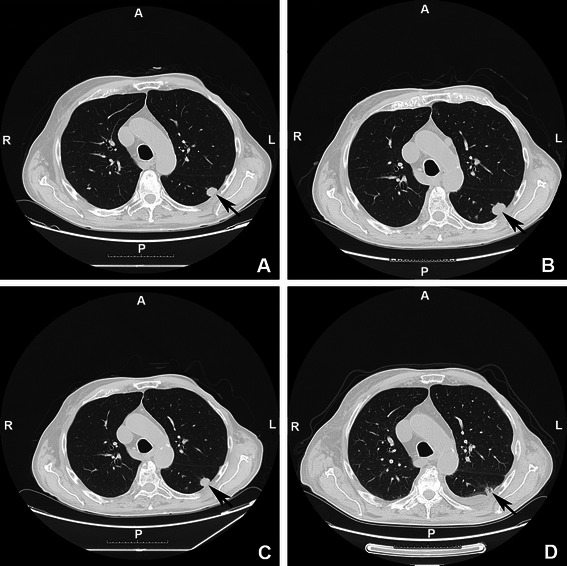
CT scan of the chest showing the radiological history of the metastatic lesion located in the apical segment of the lower lobe of the left lung. Panel a (01/2011): first evidence (maximum diameter 1.5 cm). Panel b (5/2011): dimensional increase after pegvisomant withdrawal (maximum diameter 2 cm). Panel c (09/2011): dimensional shrinkage after pegvisomant restart (maximum diameter 1.5 cm). Panel d (01/2014): further dimensional shrinkage under pegvisomant treatment (maximum diameter 1.1 cm)
